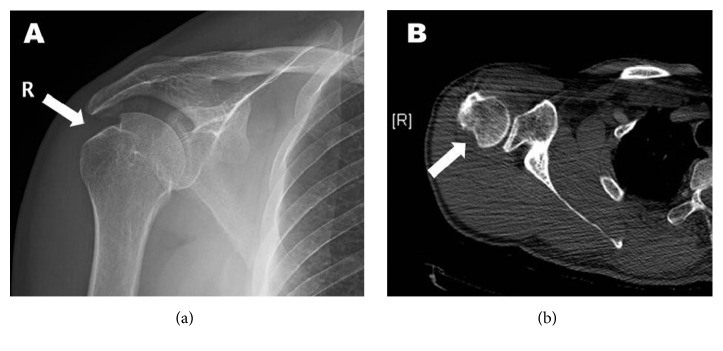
Preoperative imaging of the right shoulder. A medium size Hill-Sachs lesion is demonstrated in the posterosuperior aspect of the humeral head (arrow) in a plain AP radiograph of the shoulder (a) and in an axial plain image of a CT scan (b), suggesting previous anterior dislocation of the glenohumeral joint.
